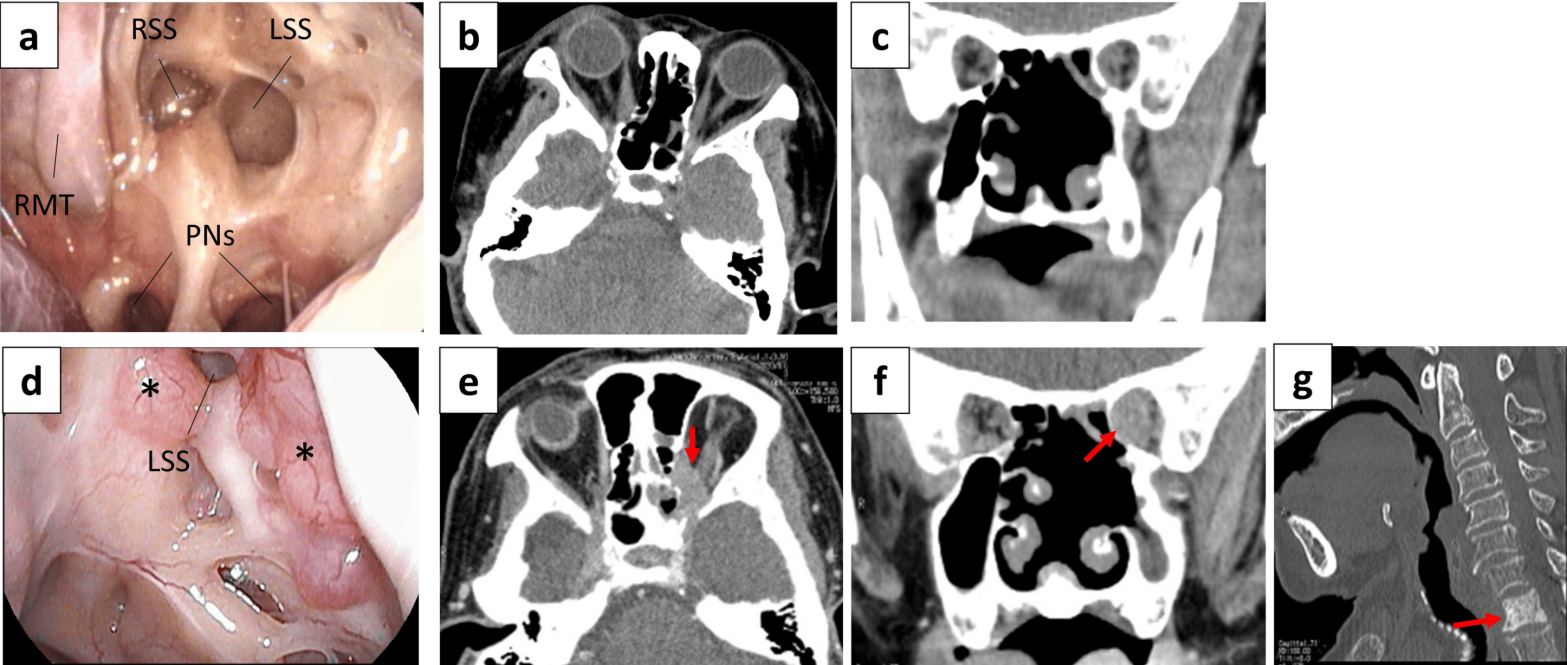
Postoperative clinical course. (a-c) Six-month follow-up with no tumor evident; (d-g) 52-month follow-up with recurrence of the nasal cavity tumor and metastasis to the 1st thoracic spine. At six months after surgery, there was no evidence of tumor in the nasal cavity (a). Axial (b) and coronal (c) CT sections did not reveal any tumor. However, at 52 months following surgery, a vascular, polypoid tumor was found in the nasal cavity (d), and axial (e), coronal (f), and sagittal spine (g). The asterisks (*) indicate the tumor. The arrows on panels e­­–g indicate recurrent tumor. RMT: right middle turbinate; RSS: right sphenoid sinus; LSS: left sphenoid sinus; PNs: posterior nostrils
